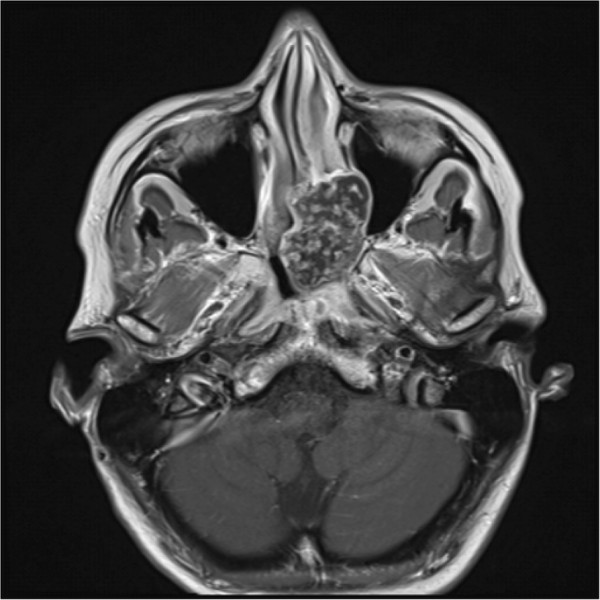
Axial T1-weighted magnetic resonance imaging scan with gadolinium of the skull showing the tumorous lesion in the left nasal cavity with infiltration of the surrounding structures.
